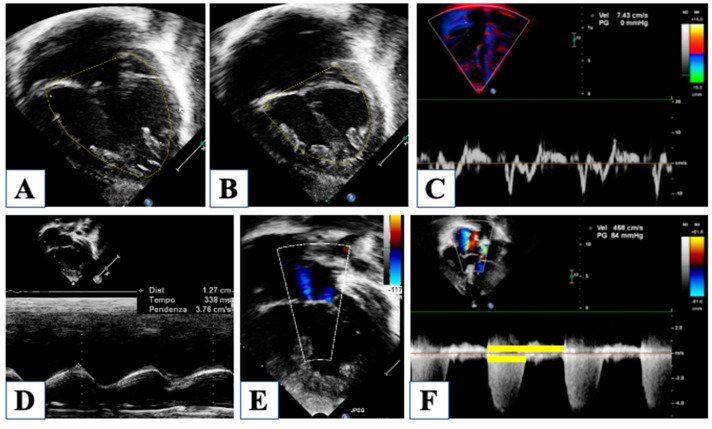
Evaluation of right ventricular function. Fractional area change of the right ventricle is obtained by tracing the endocardial border in diastole (panel (A)) and in systole (panel (B)). Tissue Doppler imaging of the lateral tricuspid annulus allows the measurement of the annular systolic velocity (panel (C)). Tricuspid annular plane systolic excursion is obtained by M-Mode (Panel (D)). Mild tricuspid regurgitation (panel (E)). Continous-wave Doppler of the tricuspid regurgitation jet allows measurement of the systolic to diastolic duration ratio (Panel (F)).
